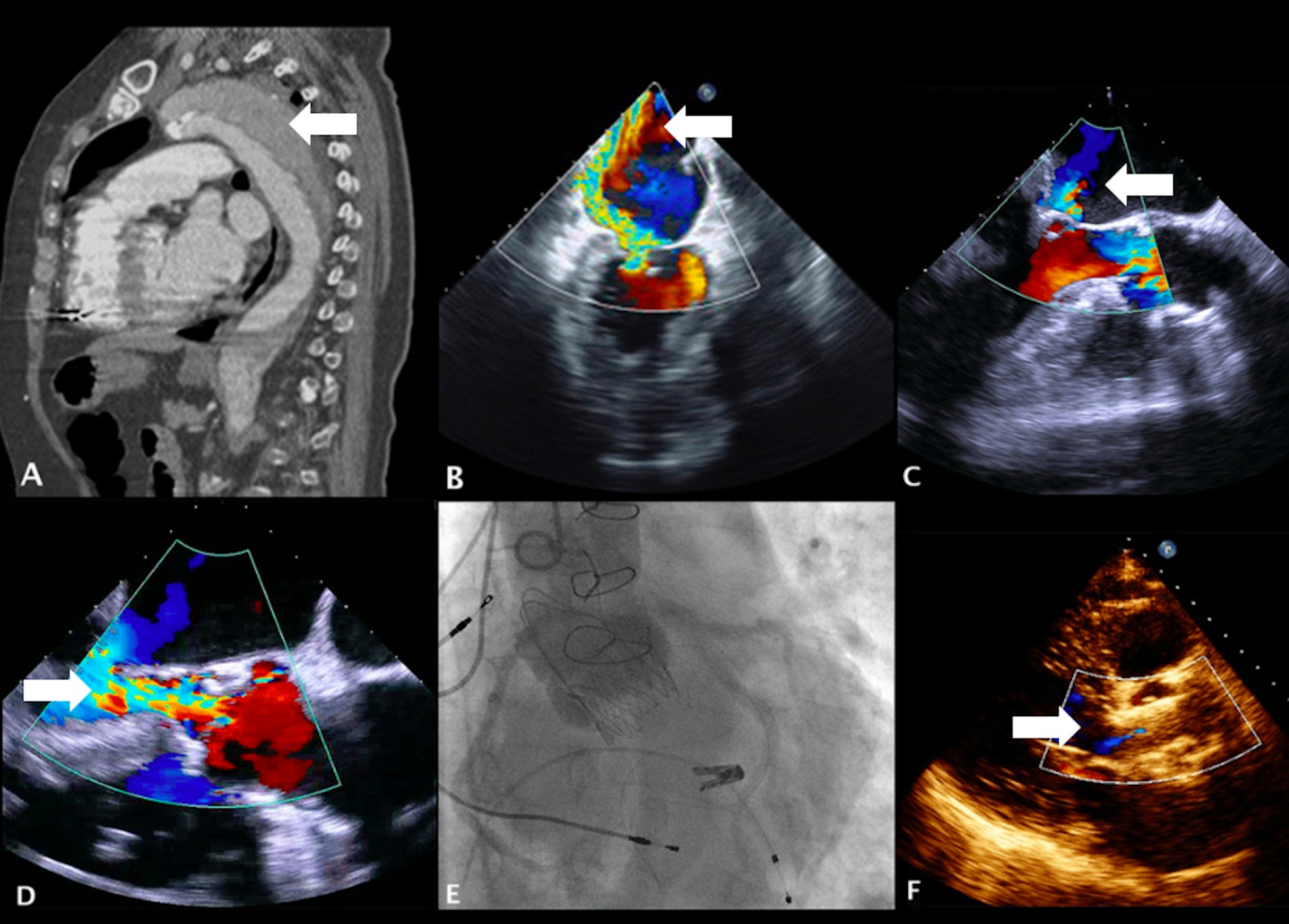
Sequential percutaneous approach for severe mitral and aortic valve regurgitation: pre- and post-procedural evaluation. (A) Sagittal reconstructed computed tomography image of the aorta, showing chronic dissection of thoracic aorta (white arrow). (B) Transesophageal echocardiography with eccentric severe mitral regurgitation reaching left atrial roof (white arrow). (C) Significant improvement of mitral regurgitation after percutaneous mitral valve repair (white arrow). (D) Severe aortic regurgitation before transapical aortic valve implantation (white arrow). (E) Final result of transcatheter aortic valve implantation, without stenosis, regurgitation or coronary obstruction (mean gradient 11 mmHg). (F) Mild aortic paravalvular leak in six-month follow-up transthoracic echocardiography (white arrow).
